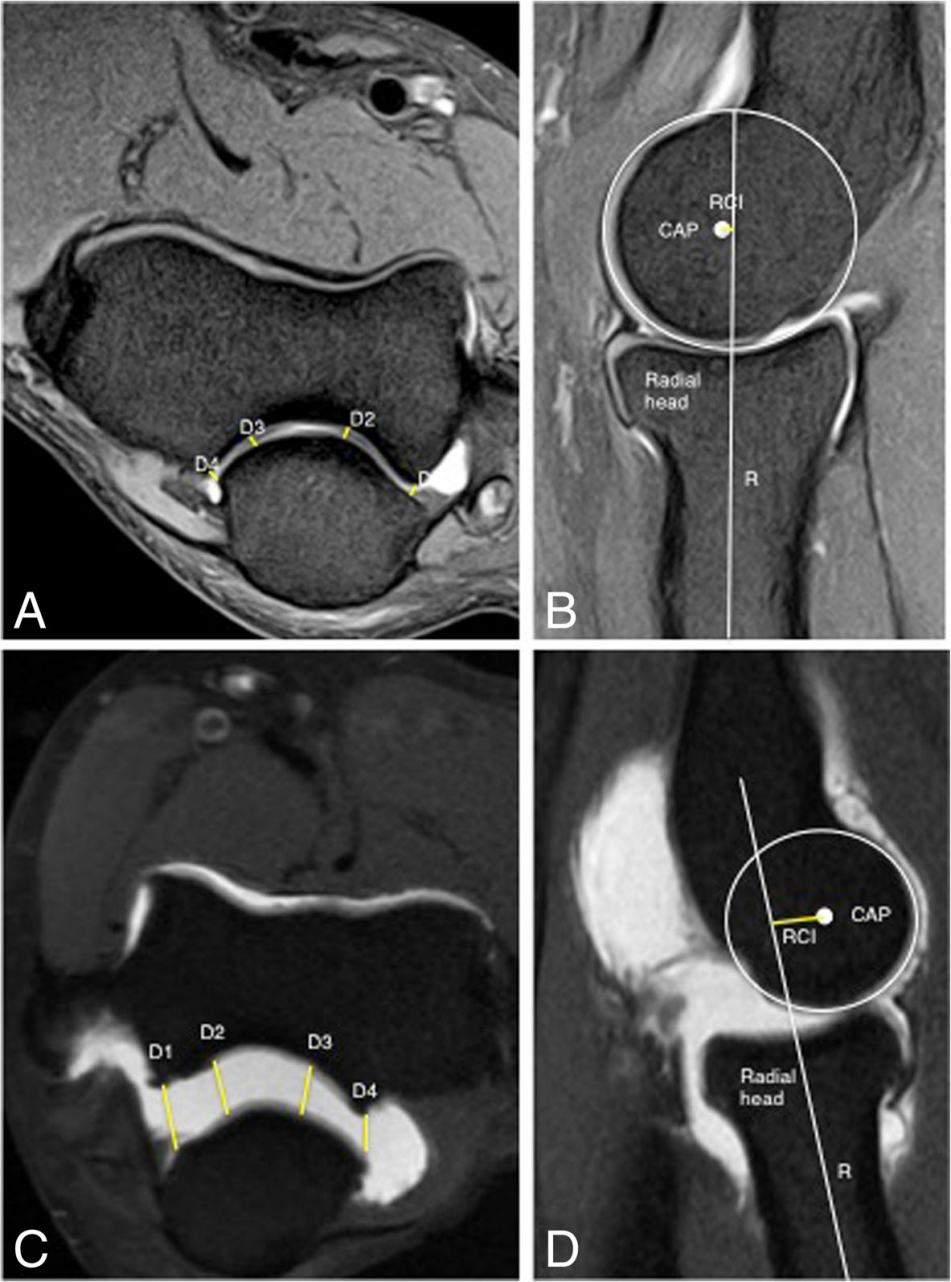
Axial FS PD-weighted MRI (a) showing the measurement of normal ulnohumeral incongruity: the distance between the trochlear joint surface and olecranon joint surface (D1, D2, D3, and D4). Sagittal FS PD-weighted MRI (b) showing the measurement of normal radiocapitellar incongruity (RCI): the distance between CAP and R. Axial FS PD-weighted MRI showing an ulnohumeral incongruity of more 1 mm (c). Sagittal FS PD-weight MRI showing a radiocapitellar incongruity of more 2 mm (d)
